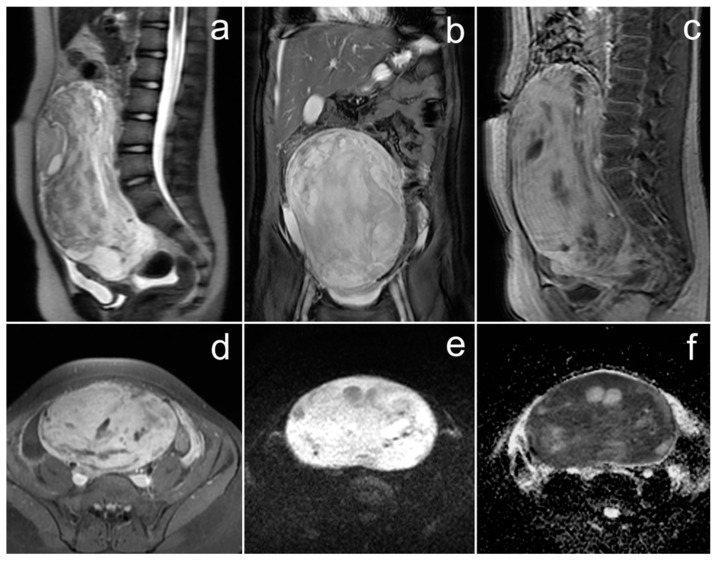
A Sertoli–Leydig cell tumor of the right ovary with rhabdomyosarcoma elements on MRI (a–f). (a)—FSE/T2; (b)—FIESTA; (c,d)—post-contrast LAVA/T1 WATER; (e)—DWI; (f)—ADC map.
